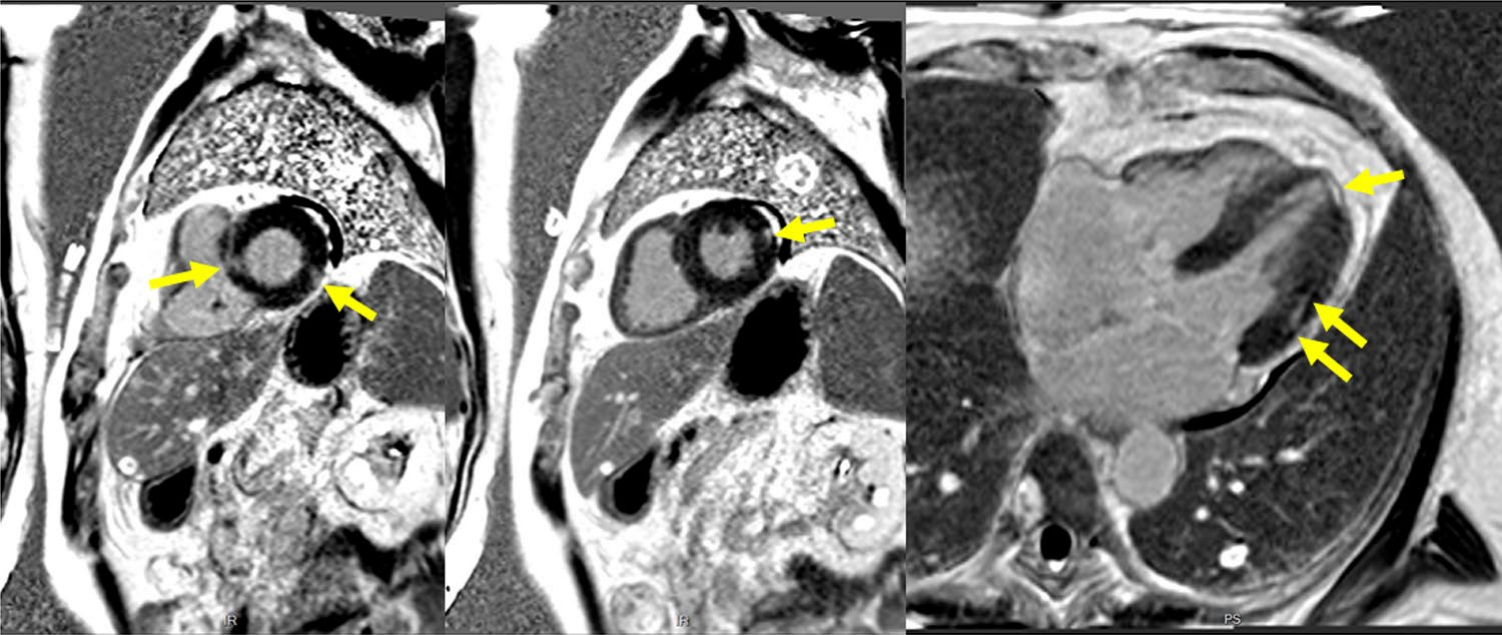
Cardiac MRI, patient 4. Late gadolinium enhancement images obtained with cardiac magnetic resonance in basal and mid-ventricular short axis orientation, and long-axis four-chamber view in a patient with ICI-induced myocarditis. Myocardial enhancement is detected in basal septal, basal inferolateral, mid anterolateral and apical segments with a sub-epicardial distribution (arrows)
